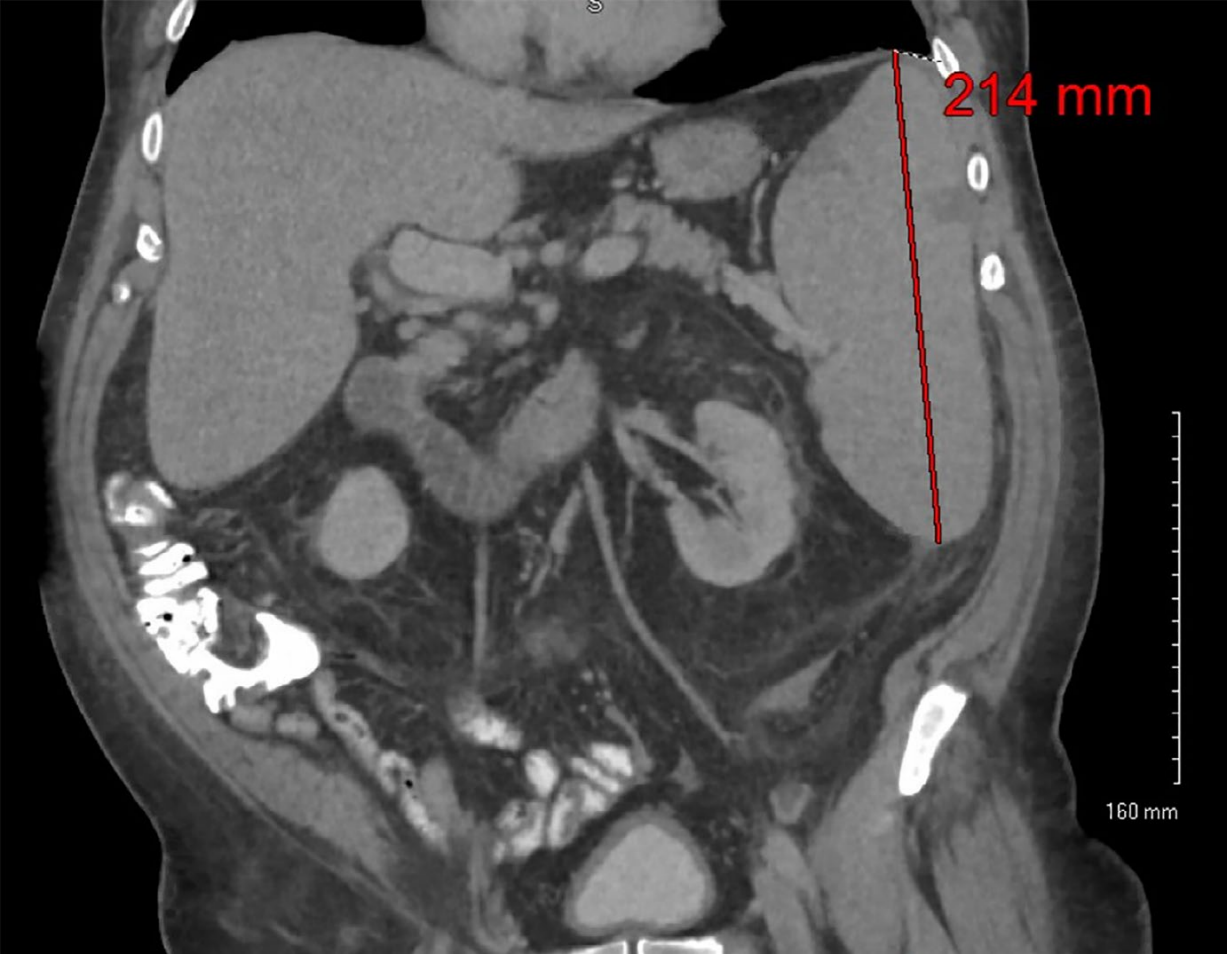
CT Abdomen/Pelvis from diagnosis, revealing splenomegaly without appreciable additional mesenteric adenopathy
